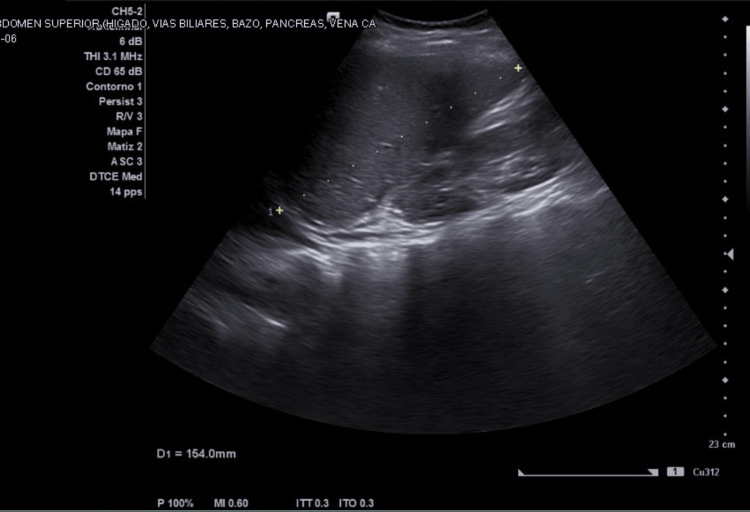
Abdominal ultrasound demonstrating hepatomegaly with increased liver span (154 mm), consistent with hepatic involvement in dengue infection.
